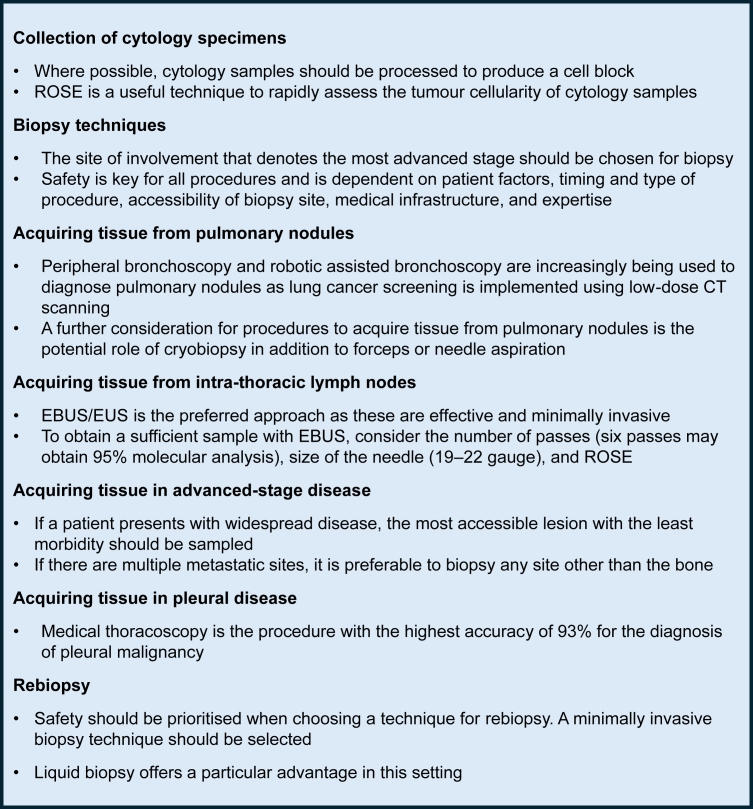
Summary of key consensus recommendations. CT, computed tomography; EBUS, endobronchial ultrasound; EUS, endoscopic ultrasound; ROSE, rapid on-site evaluation.
